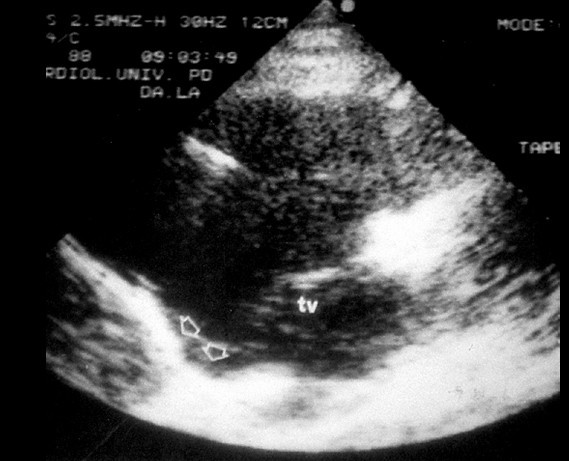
Two dimensional echocardiography findings in ARVC/D: note the presence of a typical inferior subtricuspid bulging (TV= tricuspid valve, parasternal long axis view of the RV).
